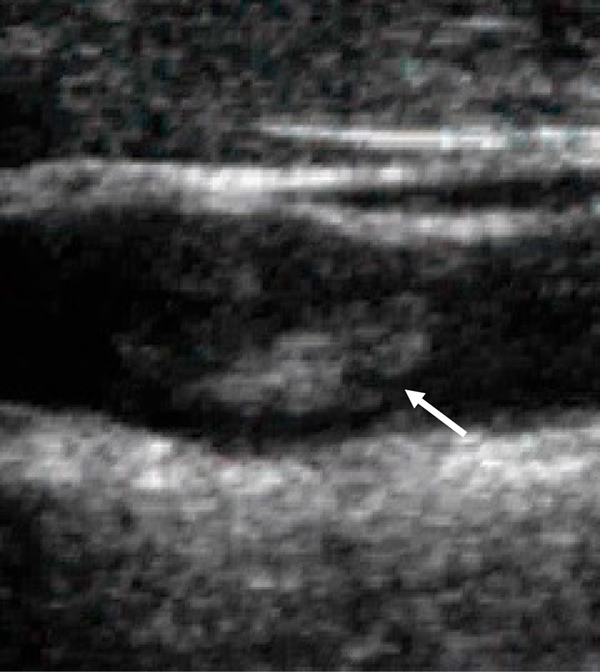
Carotid duplex sonography performed on 1 October 2003. The floating thrombus is observed at the left internal carotid artery (arrow).
